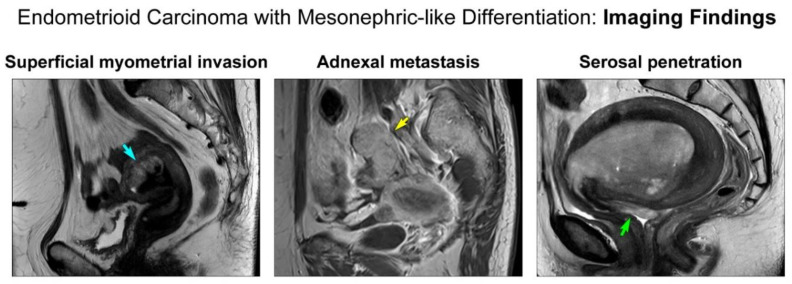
Preoperative magnetic resonance imaging findings. (Left panel) The tumor invades less than half of the myometrium (blue arrow). (Middle panel) The adnexa shows a metastatic tumor mass (yellow arrow). (Right panel) The tumor penetrates the uterine serosa (green arrow).
